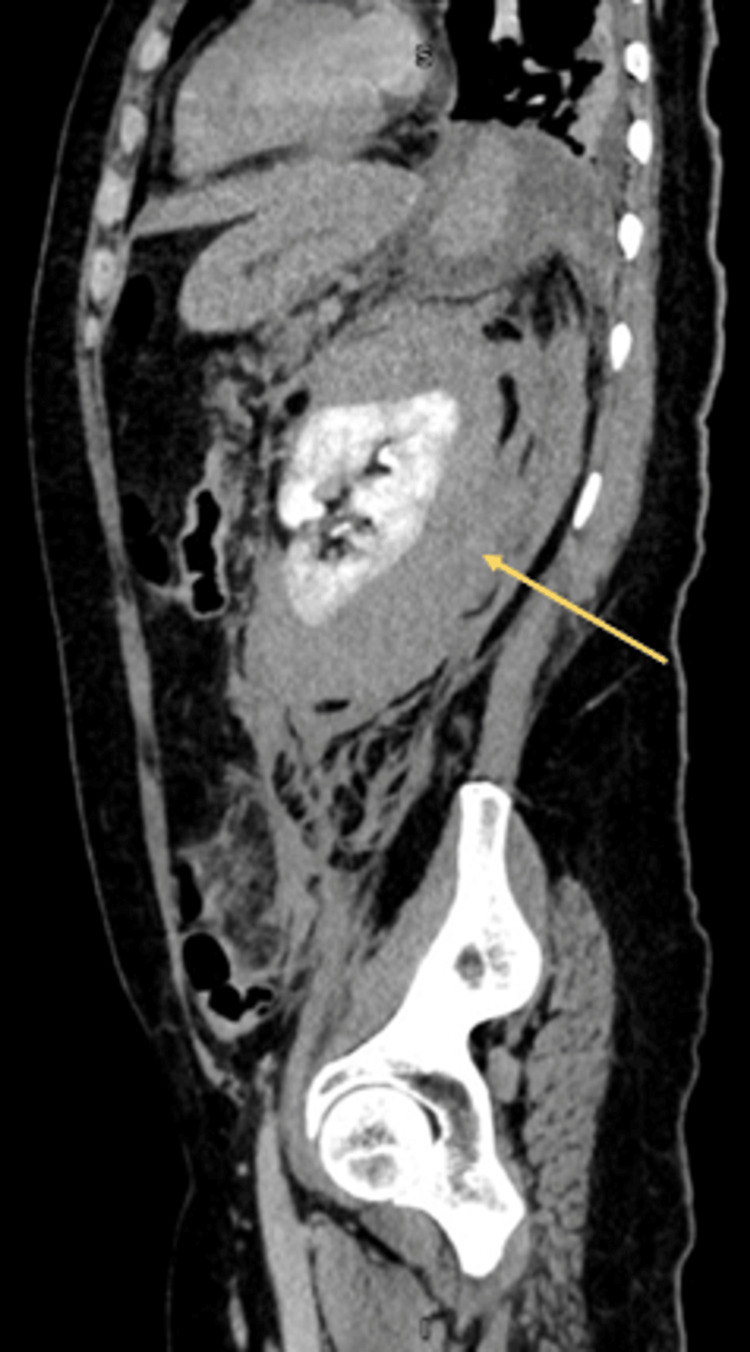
Contrast-enhanced abdominal CT (sagital view) Left kidney with a large hyperdense posterolateral collection (yellow arrow). Measurements approximately: 10 × 8.5 × 13.5 cm. Loss of left renal morphology. Obliteration of the perirenal fat stranding. No adjacent mass is observed.
